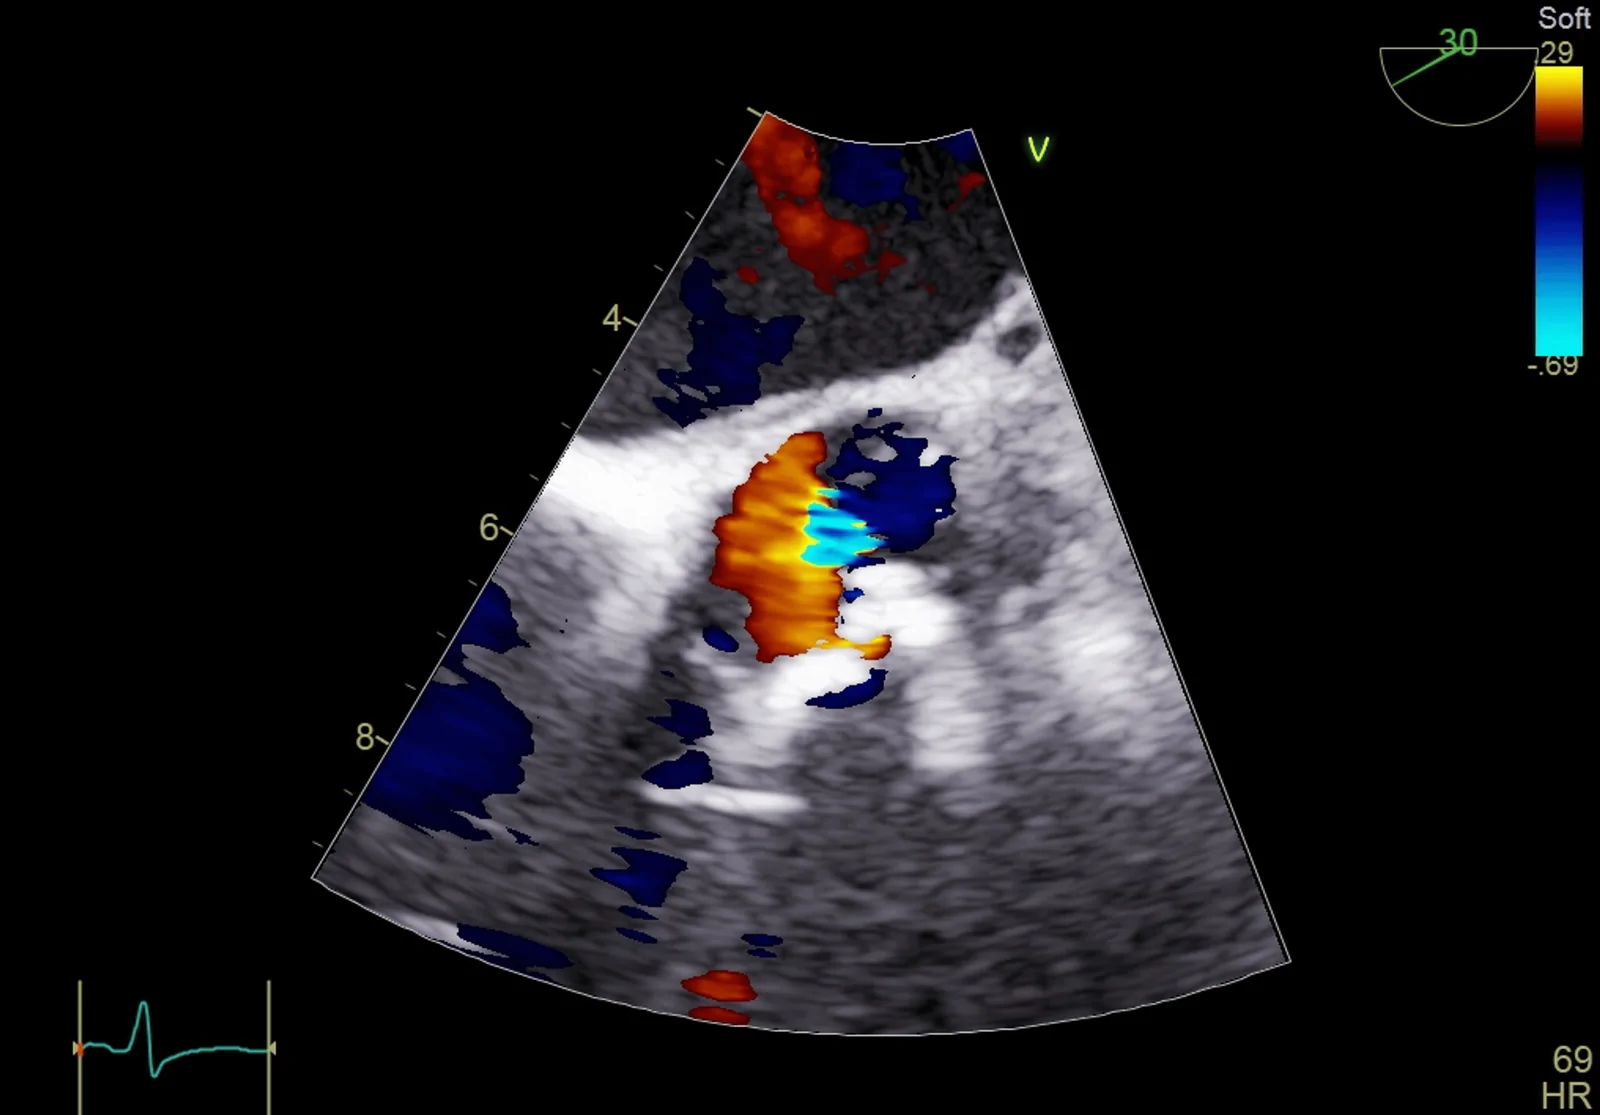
Transesophageal echocardiogram showing a short-axis view of the aortic valve (systolic, color mode). The right and left coronary cusps have fused to form a raphe (arrow). The echogenicity in this area is increased, and the areas where the color echo is not visualized along the aortic annulus correspond to the regions where the fused cusps are not neatly accommodated.
